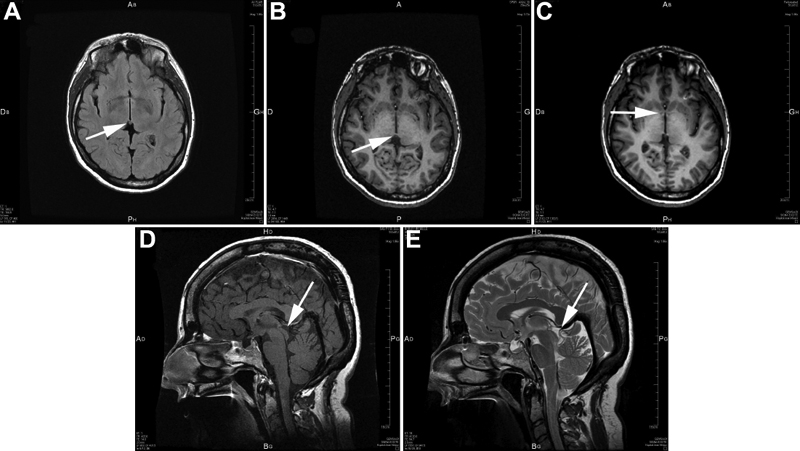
Brain MRI abnormalities in patient IV-5 from family F with the PAX6 serine 74 to glycine mutation. We show different MRI views of patient IV-5 from family F. A, B, and C correspond to MRI horizontal views, while D and E correspond to MRI sagittal views. MRI in A shows a small structure that might correspond to the habenula (arrow). MRI in B, D, and E reveals the absence of the pineal gland (arrow). MRI in C shows hypoplasia of the anterior commissure of the brain (arrow).
